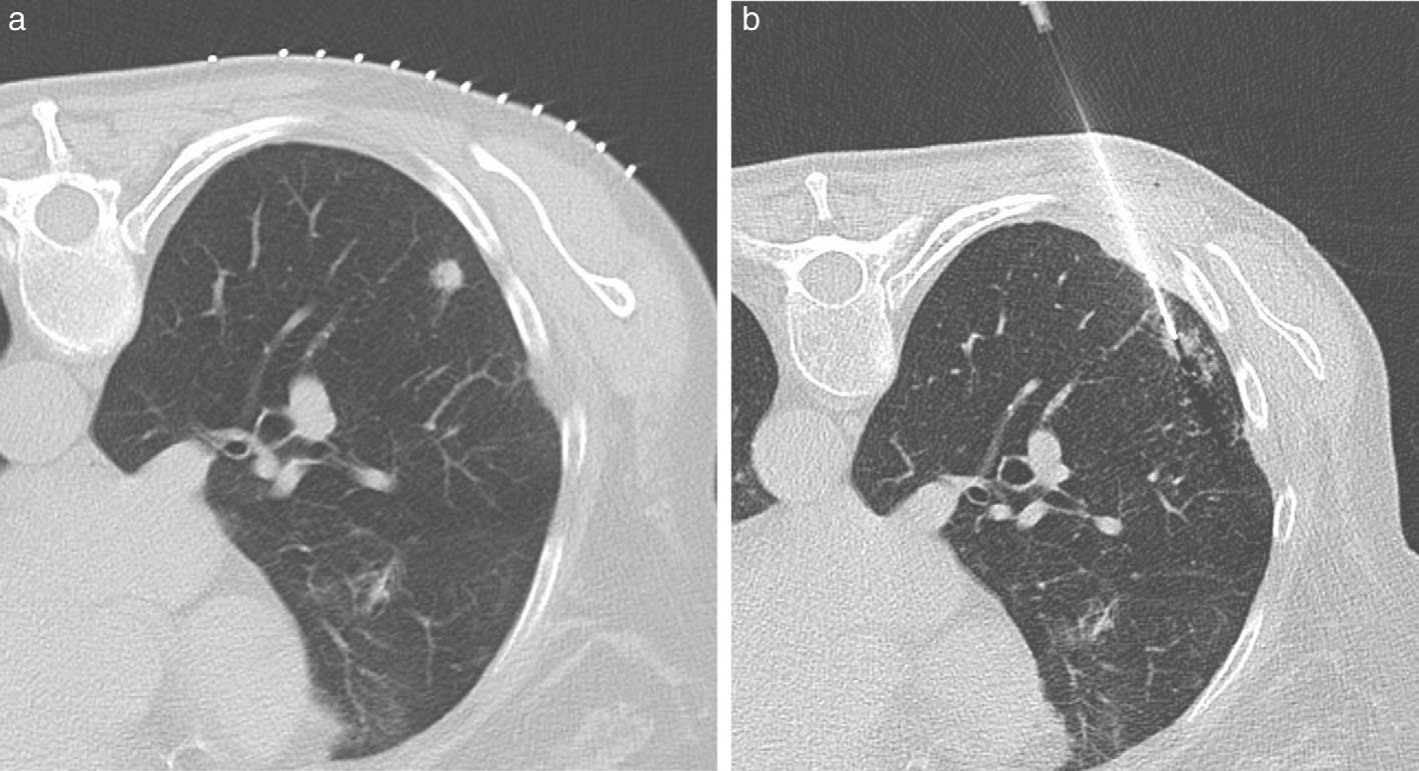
(a) Small right lower lobe solid nodule suspicious for primary lung neoplasia, in a 73‐year‐old female patient. (b) Transthoracic biopsy under local anaesthesia, followed by rapid on‐site cytological confirmation of cancer by the pathologist.
